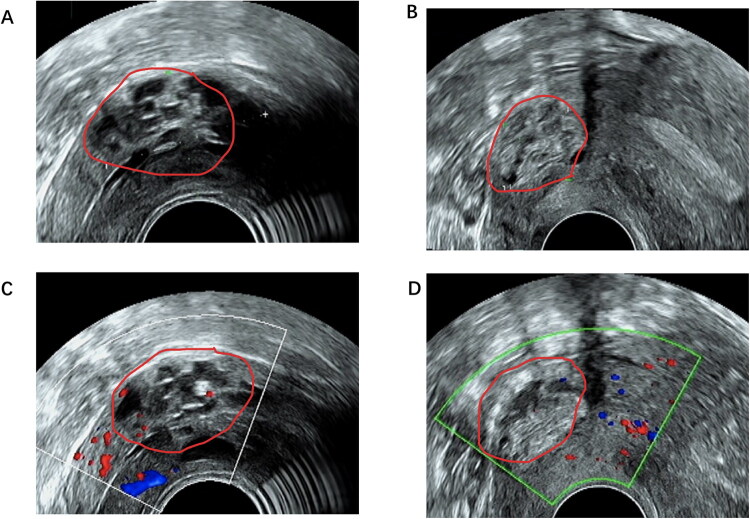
Typical transvaginal sonographic images of LEGH from different planes. A and B showed an area with uneven echoes of various size in the upper part of the cervix. The cystic lesions presented enhanced internal echogenicity and peripheral echo. And the boundary with the surrounding was not clear. C and D revealed absent or sparse blood flow signals.
